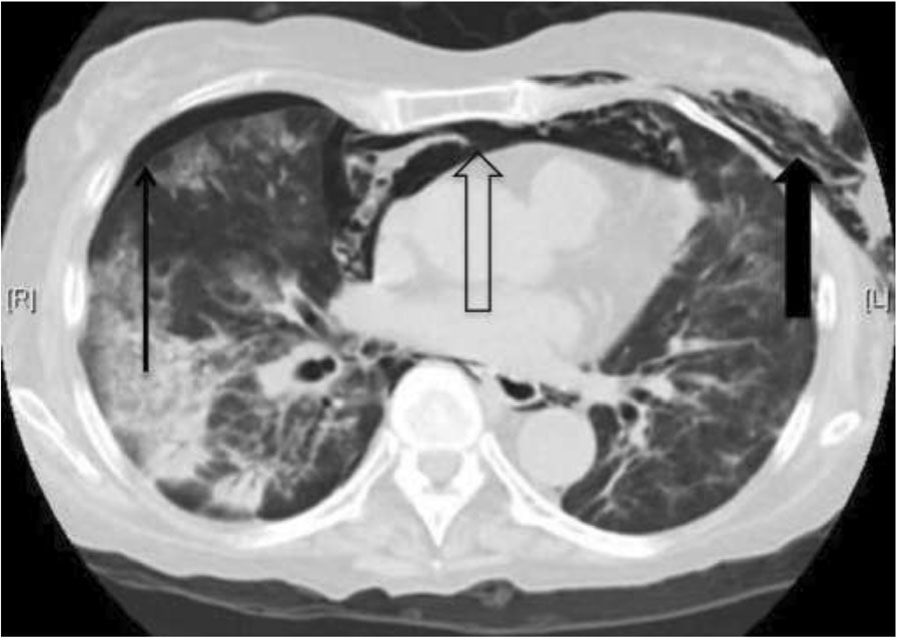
Computed tomography of thorax showing right pneumothorax (thin arrow), pneumomediastinum (hollow arrow), and subcutaneous emphysema (solid arrow)
